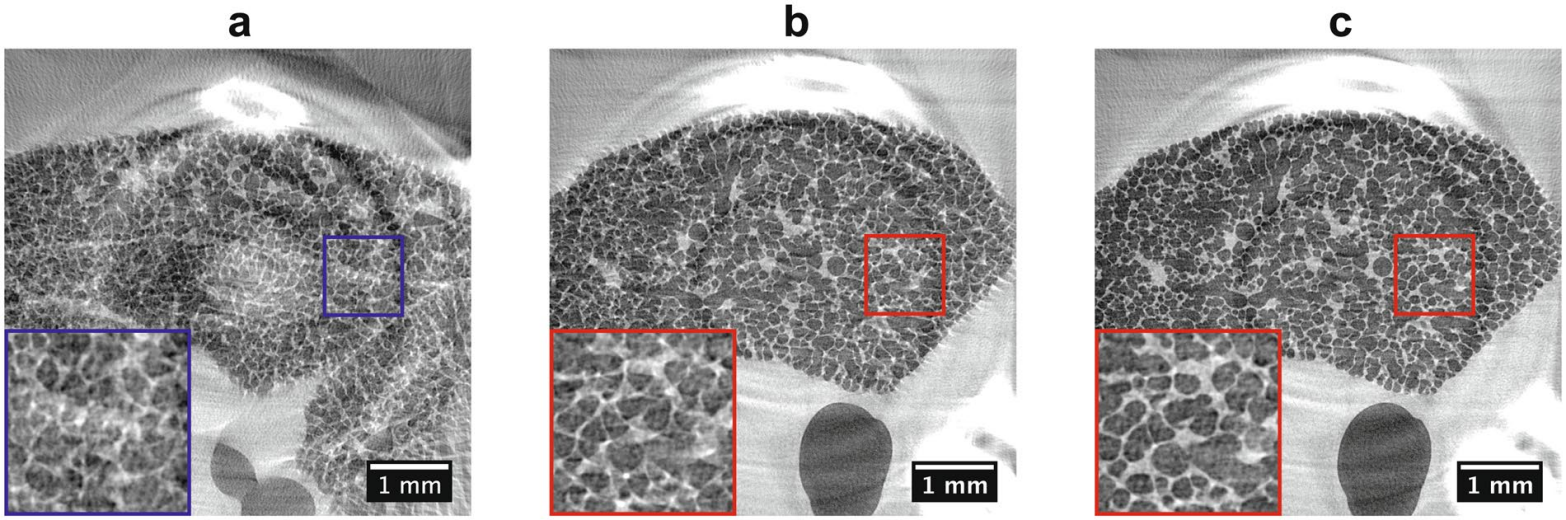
Influence of the timing of the single-projection exposure within the cardiac cycle as well as the correction for irregular heartbeat periods during a breath-hold phase under continuous rotation. Tomographic slices with approximately 450 angular projections, 2.9 × 2.9 μm^2^ pixel size, and 3 ms single-projection exposures are shown when projections are acquired at a 50 ms delay (a) and at a 150 ms delay (b) from the R-wave. Finally, since the angles of the tomographic projections are not sampled in an equiangular way, an artifact-free CT reconstruction of the same dataset (b) is obtained only after utilizing the tomographic angle of each projection in the CT reconstruction step (c).
